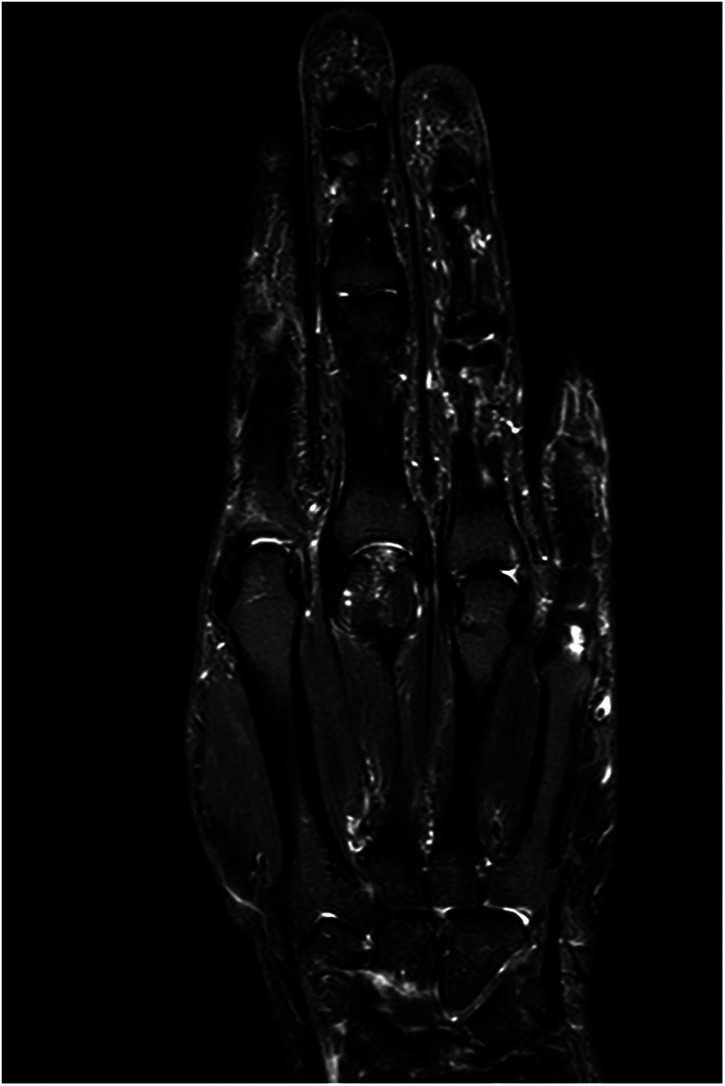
Case 1 corresponding contrast-enhanced MRI image to Figure 3 with signs of avascular necrosis.
